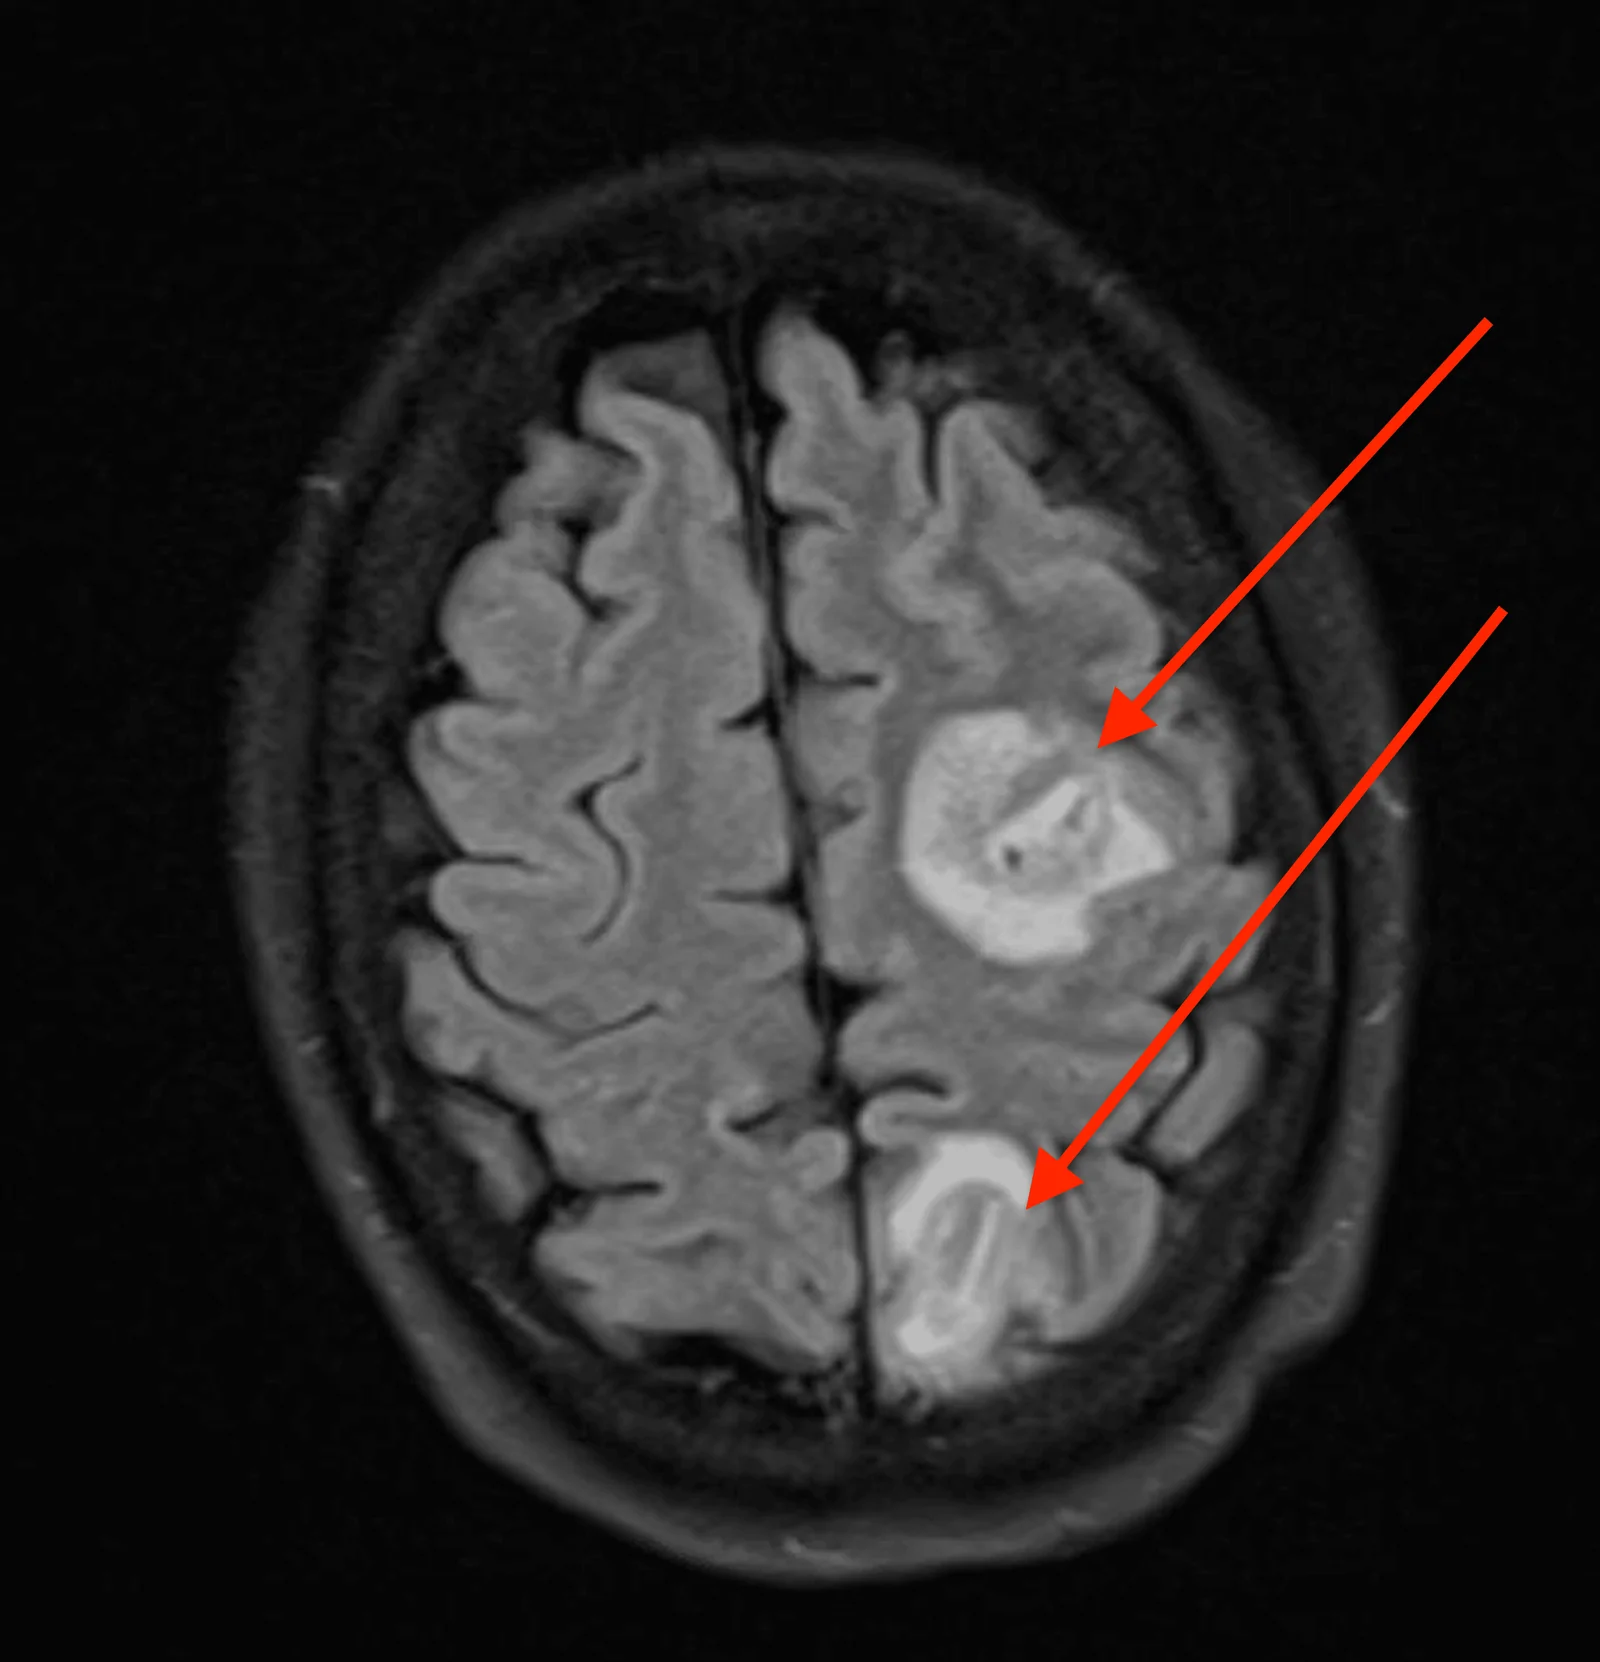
Magnetic resonance imaging (MRI) of the brain demonstrating cerebral abscesses Post-contrast axial MRI demonstrates two ring-enhancing lesions involving the left frontal (upper red arrow) and left parietal lobes (lower red arrow) with surrounding vasogenic oedema and diffusion restriction, findings favouring cerebral abscesses over metastatic disease in the clinical context of disseminated infection.
